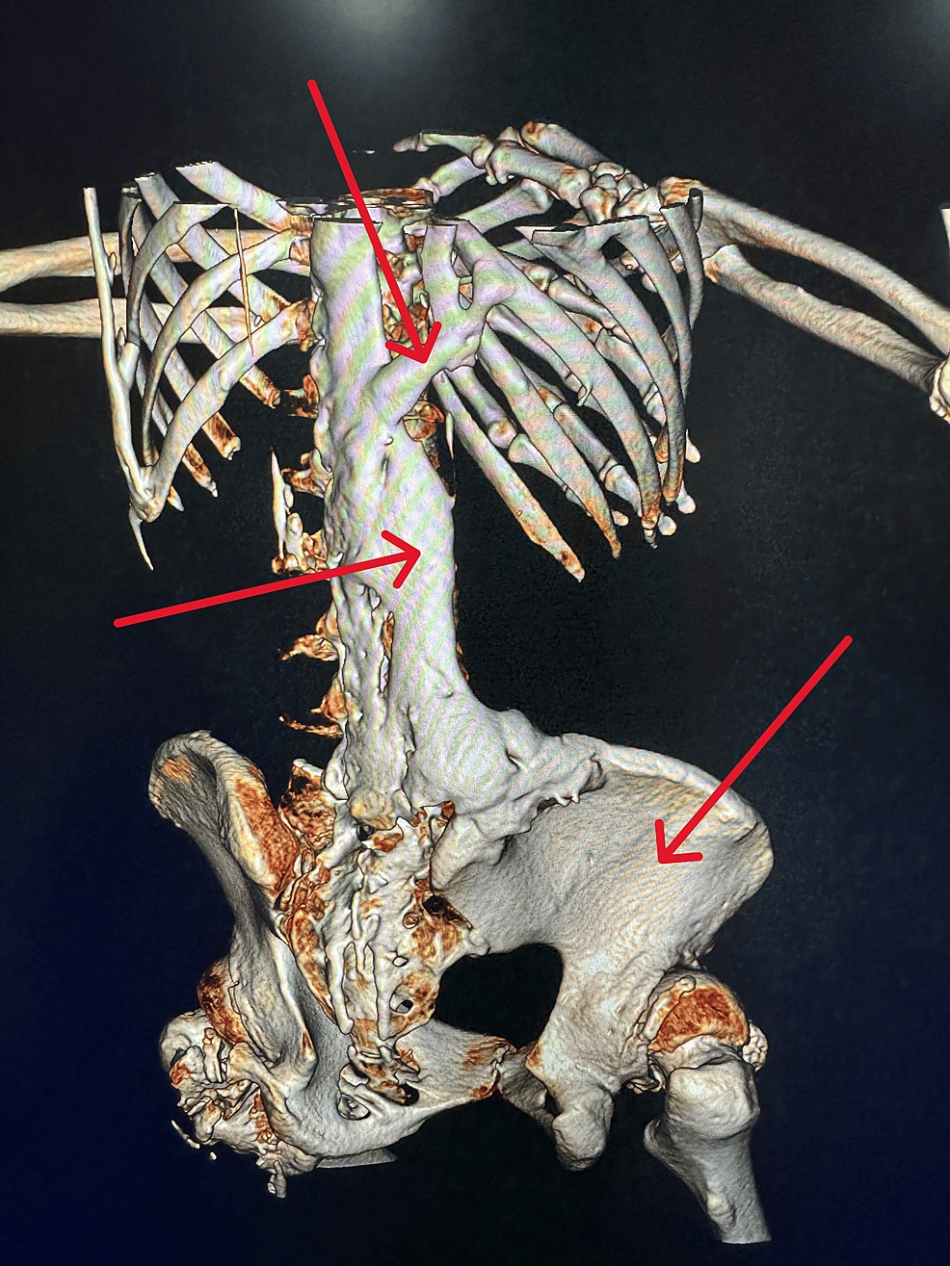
CT imaging Multiple ectopic ossification can be seen throughout the body.
